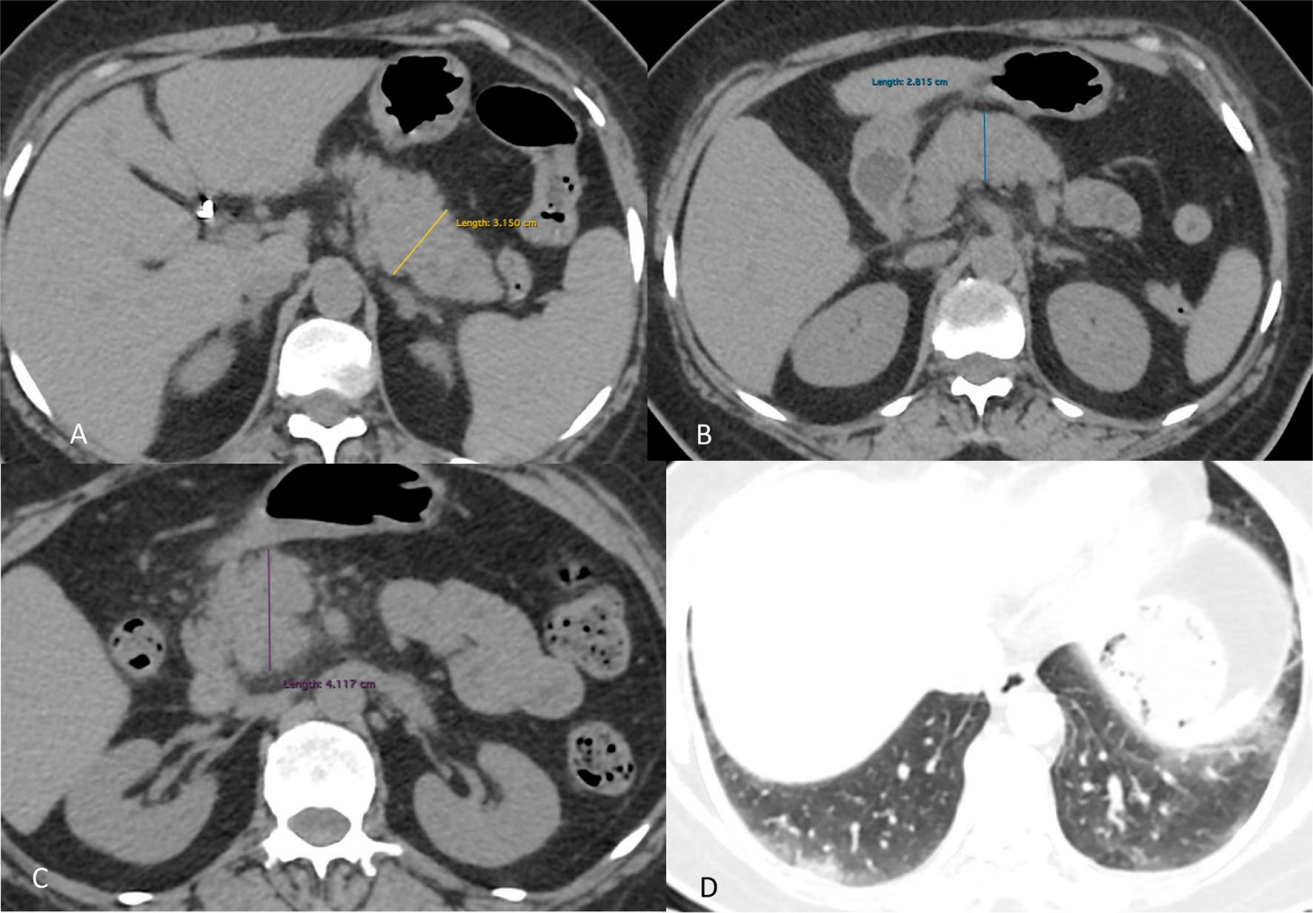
Non contrast CT images (A–C) reveal bulky pancreas with peripancreatic fat stranding. No free fluid is seen. Evidence of post cholecystectomy clips is noted in image A. D Lung window of the same patient shows COVID-19 changes in both lung fields
